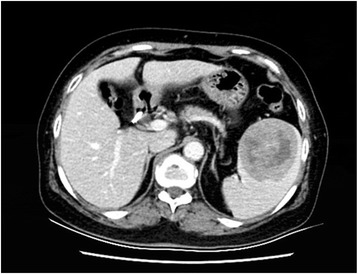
Abdominal computed tomography scan revealed a big, single hypodense, 6.4 × 6.5 cm in size lesion in the spleen. Clear boundary, heterogeneous enhancement, and centrally irregular necrosis
